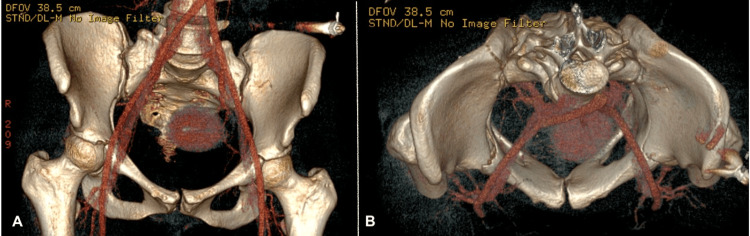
3D CT scan of bilateral acetabular fractures. (A) Anterior view of 3D CT scan showing right nondisplaced anterior, middle, and posterior acetabulum fractures and left nondisplaced anterior and middle acetabulum fractures. (B) Axial view of the pelvis from 3D CT scan.
